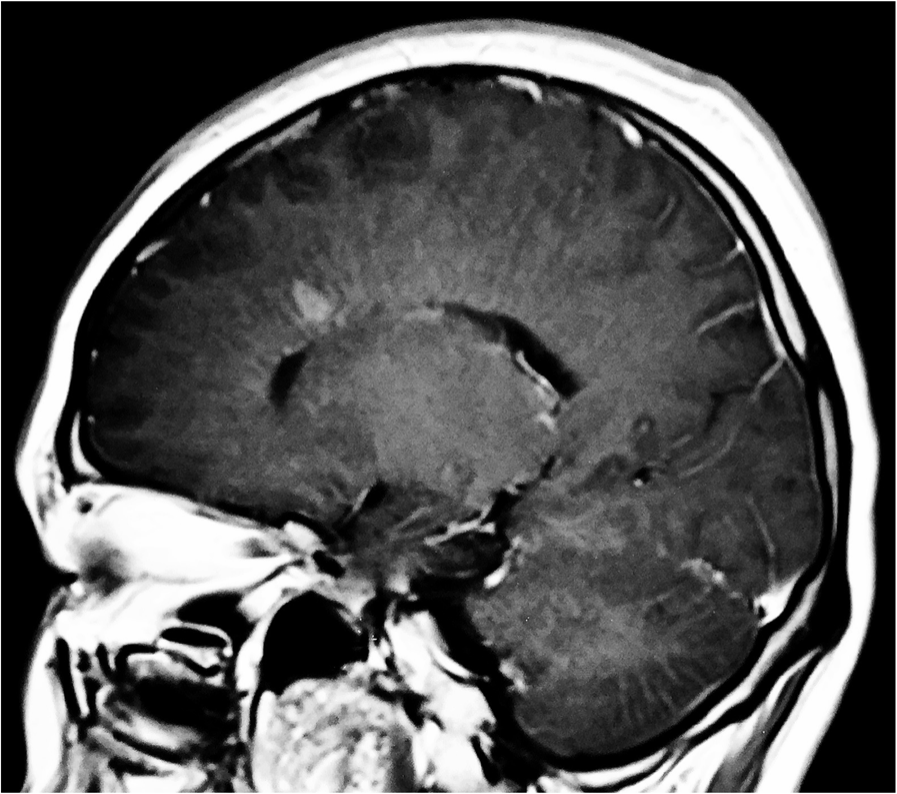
Sagittal T1 post-contrast sequence MRI showing a radial pattern of linear periventricular post-gadolinium enhancement
